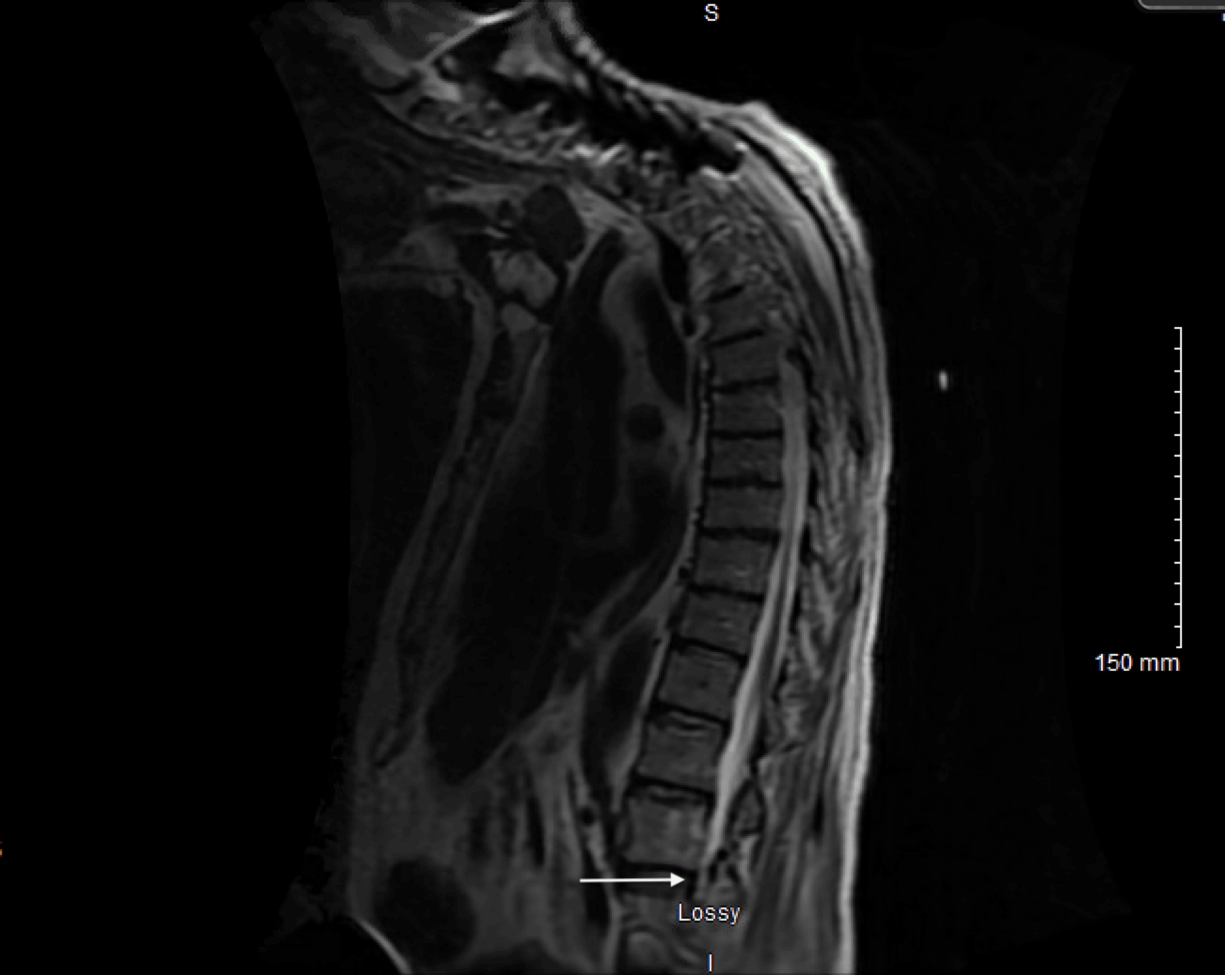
Sagittal T2-weighted MRI of the thoracic and lumbar spine with and without contrast, demonstrating mild-to-moderate foraminal narrowing from L3 to S1, consistent with chronic degenerative and inflammatory changes.
